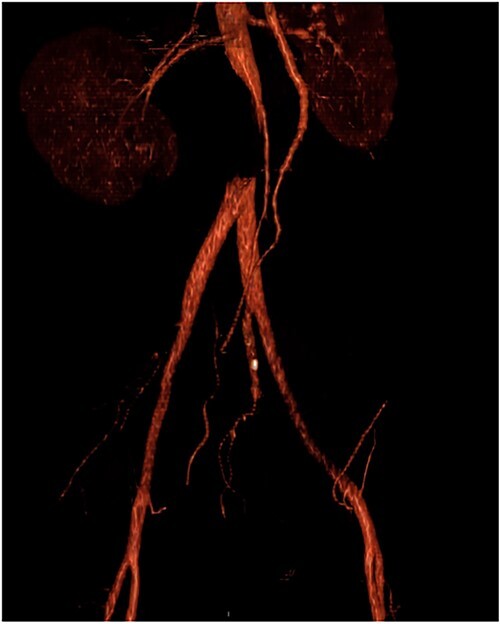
CTA with occlusion of the infrarenal abdominal aorta above the bifurcation of the iliac arteries.
